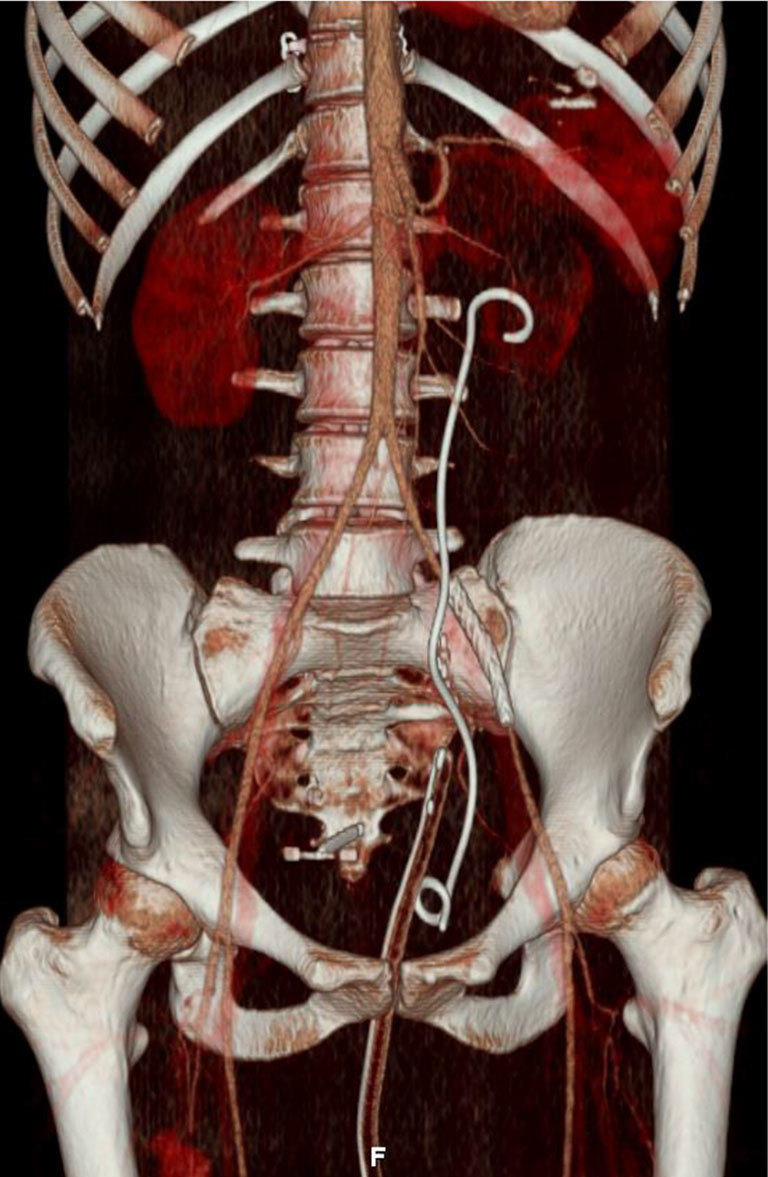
Three-dimensional arterial phase CT scan showing stent graft of the left common iliac artery aneurysm, left ureteral stent and right profunda femoris artery (prior to thrombin injection, rupture or embolization coils).
